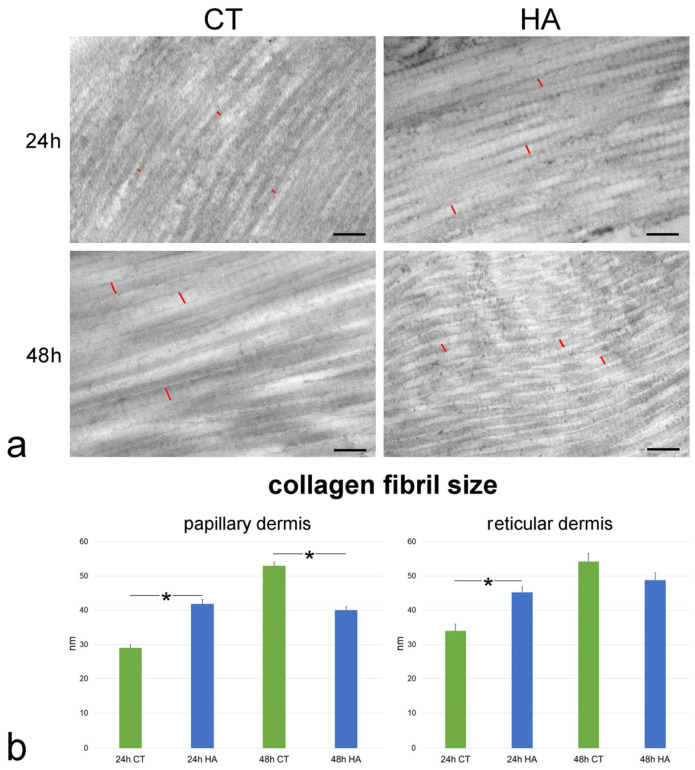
(a) TEM images of collagen fibrils in the papillary dermis of control (CT) and hyaluronic acid (HA)-treated skin. Red lines indicate the size of some collagen fibrils. Bars, 200 nm. (b) Mean ± SE values (*n* = 40) of collagen fibril size in papillary and reticular dermis of control (CT) and hyaluronic acid-treated (HA) skin samples at 24 and 48 h. The asterisks (*) indicate a statistically significant difference between HA-treated samples and the relative CT.
